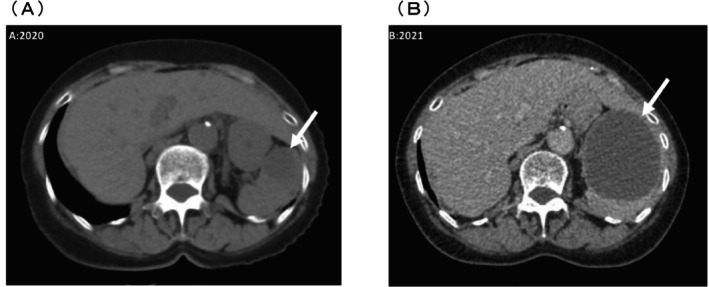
Computed tomography scans reveal a splenic mass that increased in size from 30 mm in 2020 (A) to 79 mm in 2021 (B).
